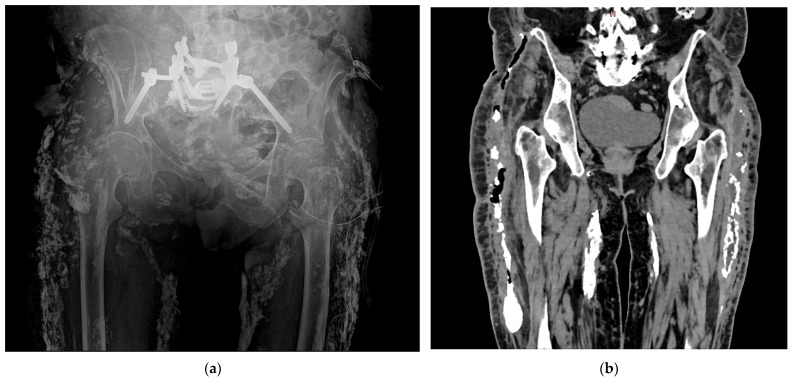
Radiologic assessment of a 65-year-old female patient with fever and known for calcinosis cutis universalis: (a) plain radiograph showing multiple calcifications along the hips; (b) further evaluation with CT scan demonstrating air adjacent to right sided calcified lesions, raising suspicion of secondary infection of calcinosis cutis universalis.
